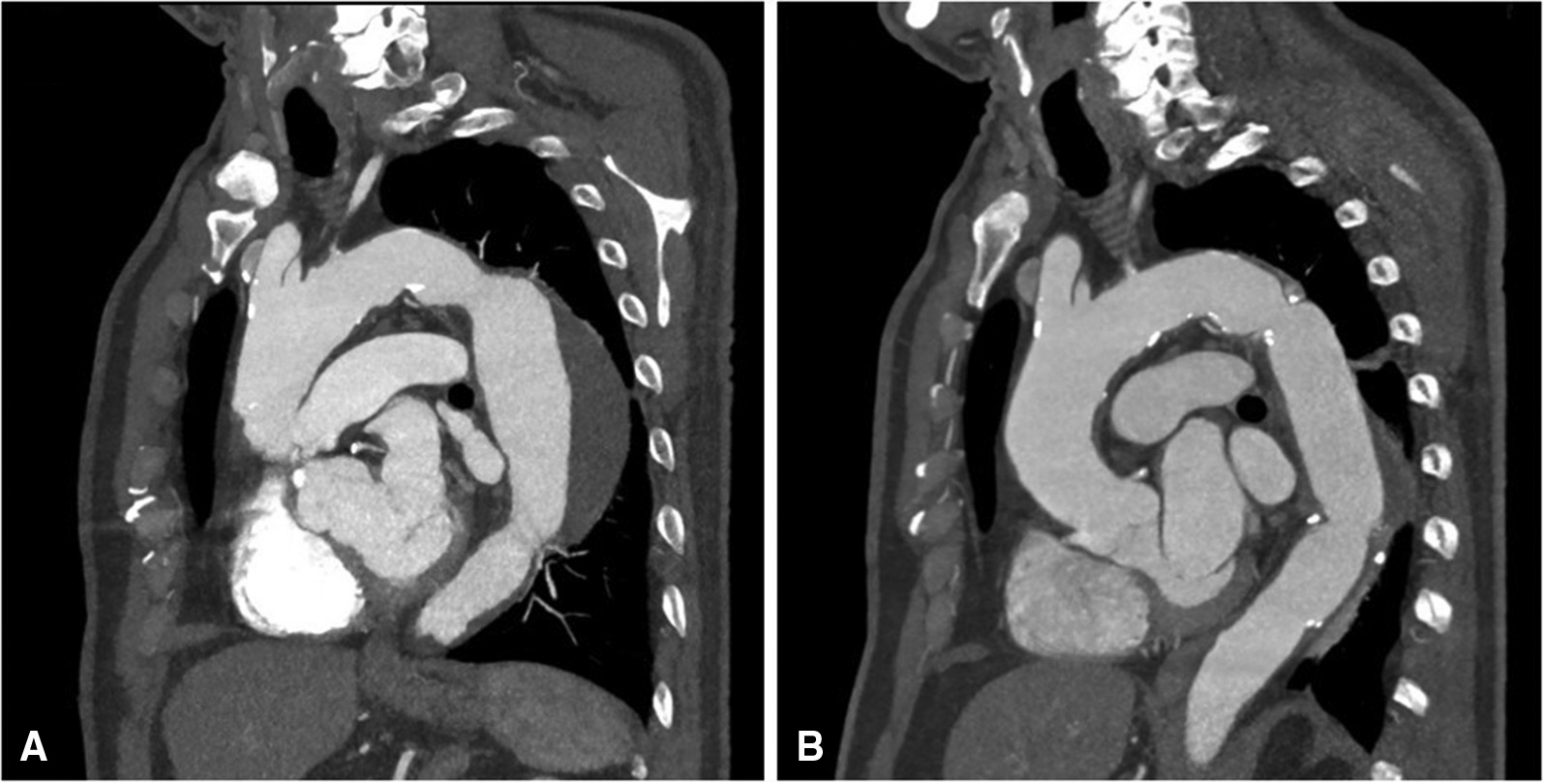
Preoperative (A) and postoperative computed tomography (CT) scans at 6 months (B) of a patient with degenerative aneurysm of 64 mm in diameter and 90 mm in length. Postoperative CT scan is routinely performed immediately after surgery during the index hospitalization and 3 to 12 months after surgery in all patients.
